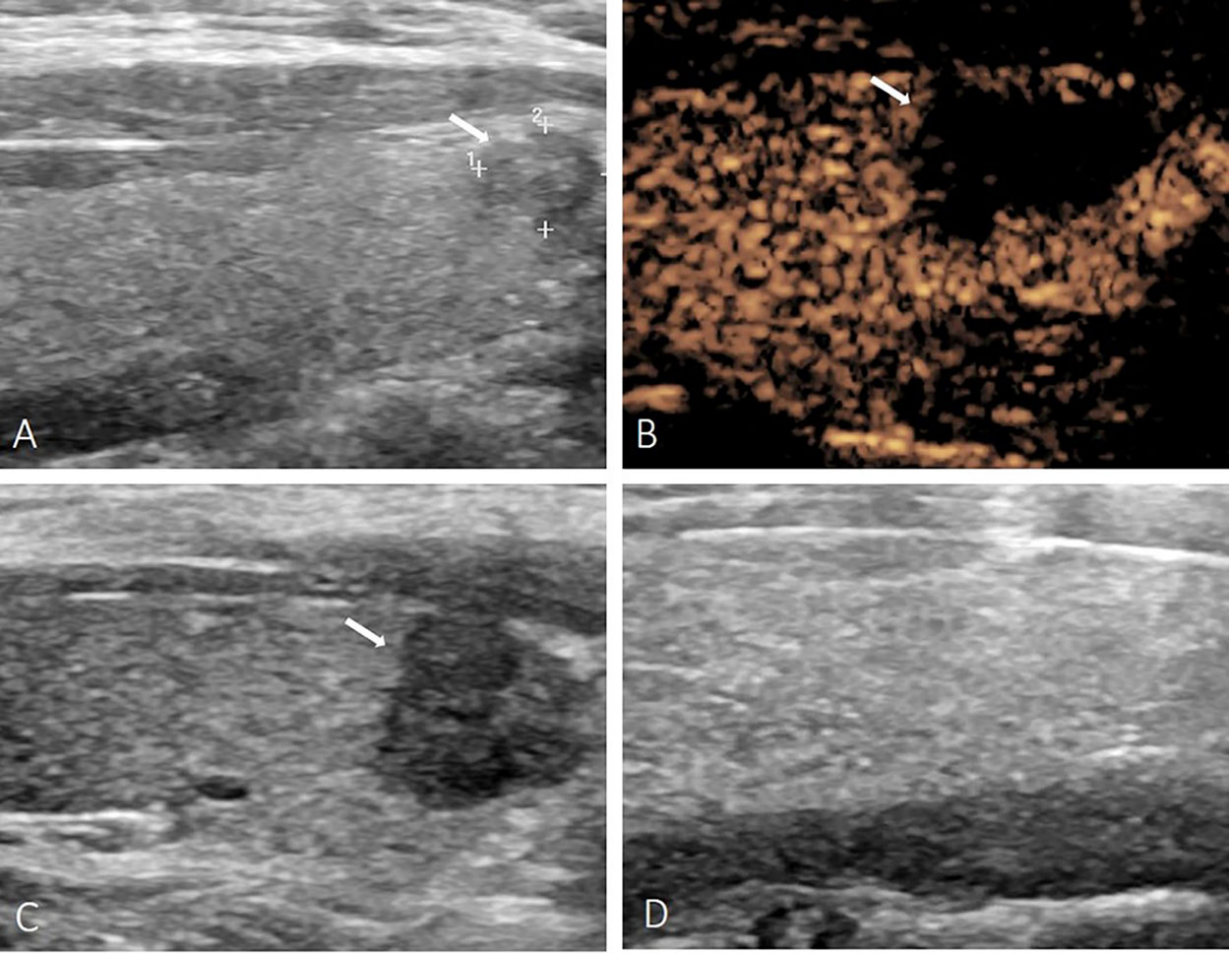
A 35-year-old female with PTMC. (A) Before MWA, US image showed a tumor (arrow) located in the right lobe. (B) At 1 day after MWA, contrast-enhanced ultrasound showed the PTMC was completely ablated. (C) At 1 month after MWA, the volume of the ablation area (arrow) was gradually reduced. (D) At 6 months after MWA, the ablation area was completely disappeared on US image.
